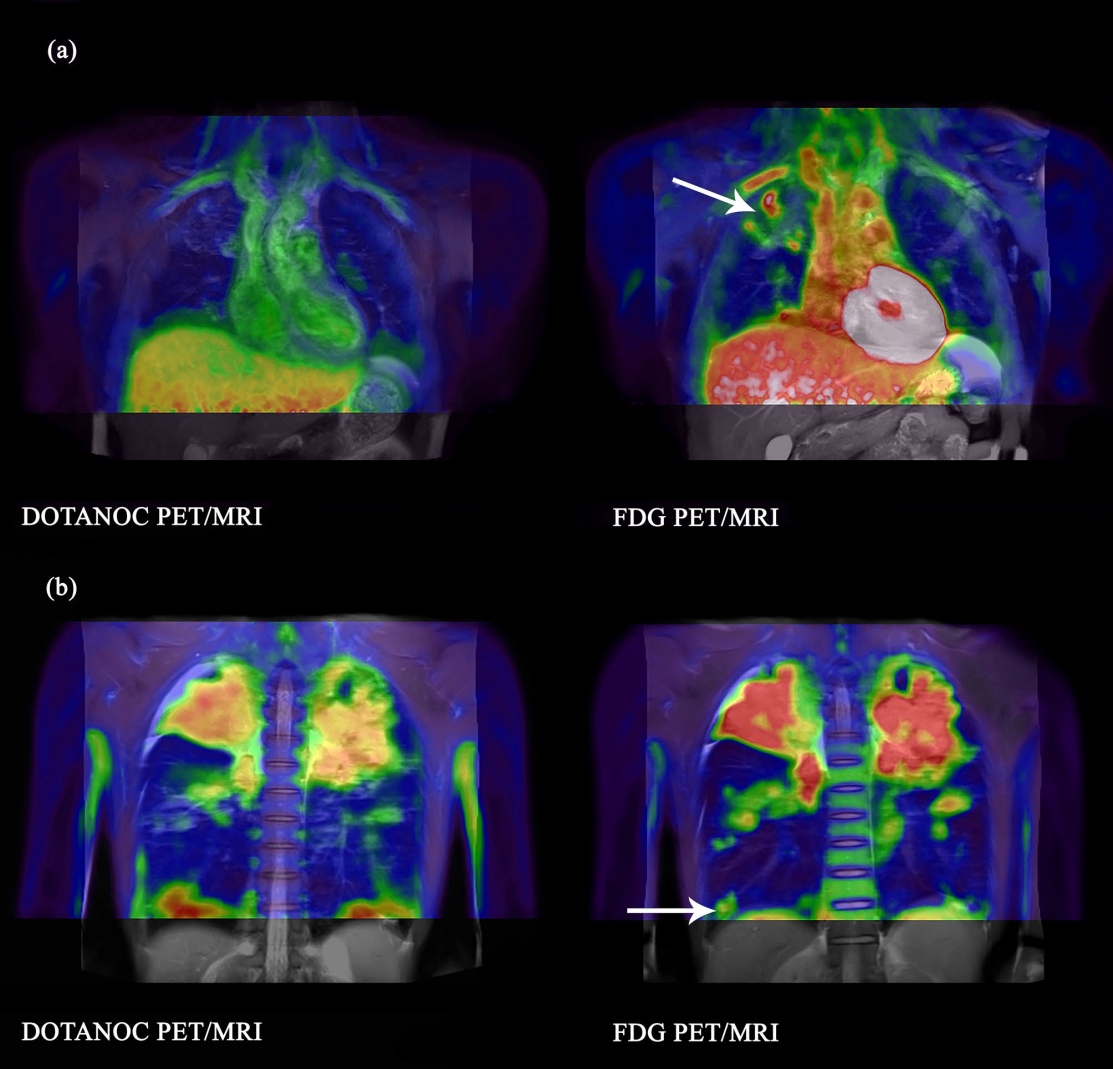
Lesions visualised with FDG but not DOTANOC in pulmonary TB (a) Lesions in right upper lobe visualised with FDG, largest volume 16.5 cm^3^, SUVmax 2.89. No uptake with DOTANOC. 22-year-old male, smear 2+, on TB treatment for 28 days. (b) Lesion in posterior basal segment of right lower lobe visualised with FDG, volume 4.3 cm^3^, SUVmax 2.50. No uptake with DOTANOC. 29-year-old male, smear 3+, on TB treatment for 25 days.
